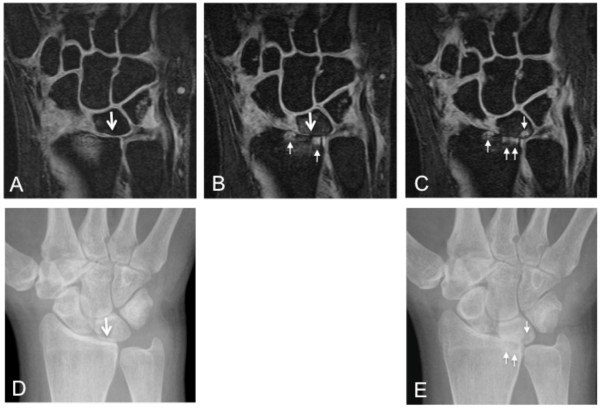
Progression of joint damage in the radiolunate joint. Magnetic resonance imaging (MRI) of the wrist shows thin but present high-signal-intensity cartilage over articular surfaces of the radiolunate joint (large arrow) at baseline (A) associated with grade-1.0 cartilage thinning. Corresponding baseline conventional radiography (XR) (D) shows joint-space narrowing (JSN) at this location (large arrow). Follow-up MRI at 12 weeks (B) and 24 weeks (C) show progressive JSN associated with loss of cartilage over both articular surfaces of this joint, indicative of grade-3.0 cartilage loss. Note development and progression of bone erosions (small arrows) in the radius and lunate on these follow-up scans. Week-24 XR also shows complete radiolunate JSN, the lunate erosion and two of the radius erosions (small arrows). However, the third erosion in the radius is not visible on XR.
